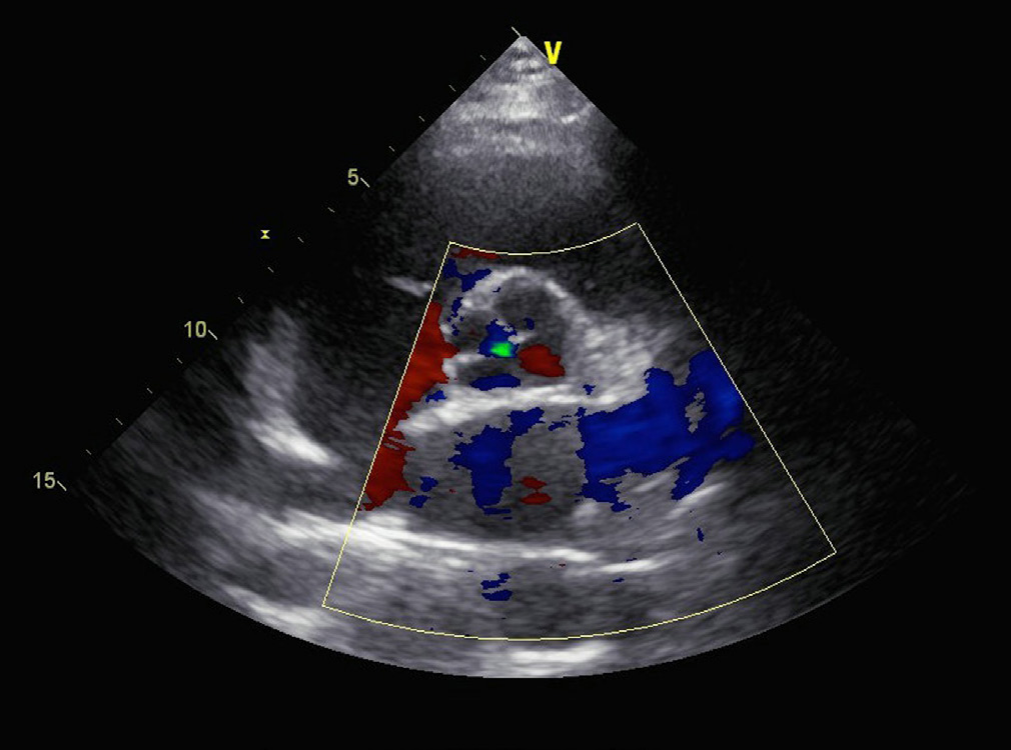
Echocardiography of a type D/type III quadricuspid aortic valve with mild
